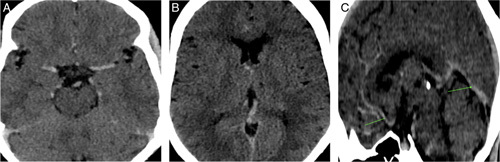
(A) Non-contrast axial computed tomography (CT) image at the level of midbrain shows the hyperdense bilateral middle cerebral arteries. (B) Non-contrast axial CT image at the level of basal ganglia shows the hyperdense bilateral internal cerebral veins (left>right). (C) Non-contrast midsagittal CT image showing hyperdense straight sinus, great cerebral vein (green arrow) and anterior cerebral artery (green line).
